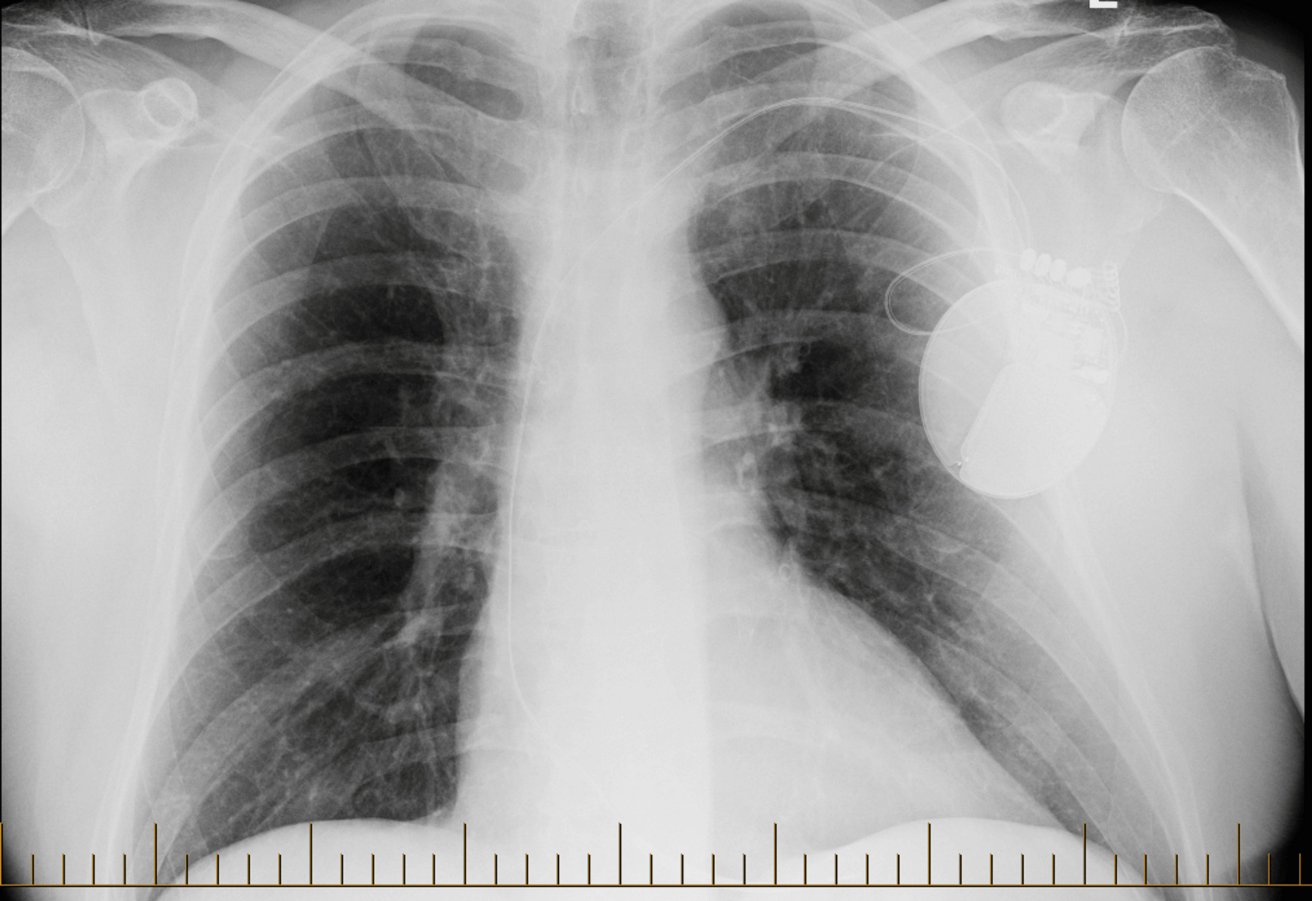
Posteroanterior (PA) chest X-ray showing no acute cardiopulmonary findings The imaging demonstrates a single-lead left subclavian implantable cardioverter-defibrillator (ICD) in place, with stable positioning and no signs of lead displacement or complications.
